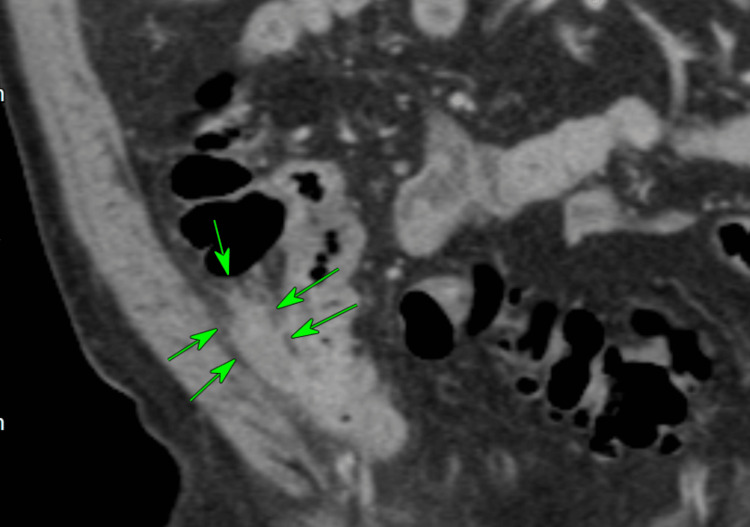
CT Scan coronal view showing thickened appendix associated with peri-appendiceal inflammatory changes within the RIF, consistent with acute appendicitis. RIF: right iliac fossa
